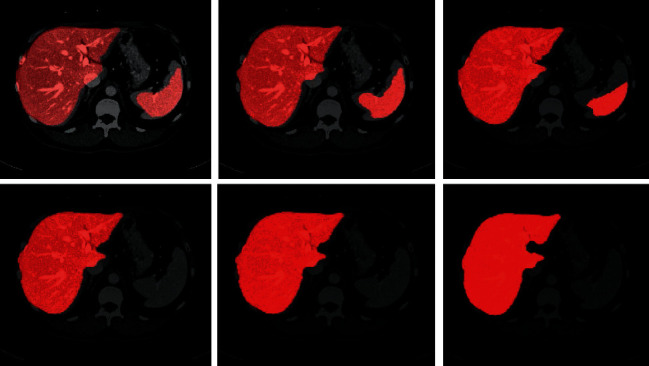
Iterative results of liver probability map produced by Ga-CNN model on CT scan image of SLiver'07 dataset. From top left to bottom right, 8^th^, 15^th^, 25^th^, 55^th^, 60^th^, and 70^th^ iterative liver probability maps are shown. The brighter region shows the more probability map of the liver.
